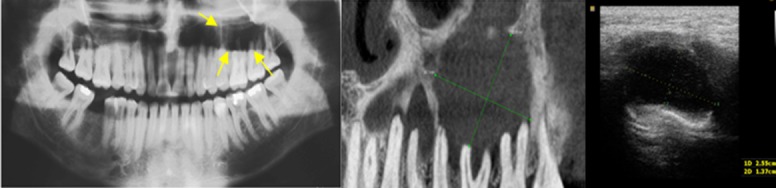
A case of odontogenic keratocyst. (A) Panoramic view reveals a radiolucent lesion in left posterior region of the maxilla. (B) CBCT shows lytic lesion and a prominent septum inside the lesion. (C) Ultrasound image shows a well demarcated hypoechoic lesion.
